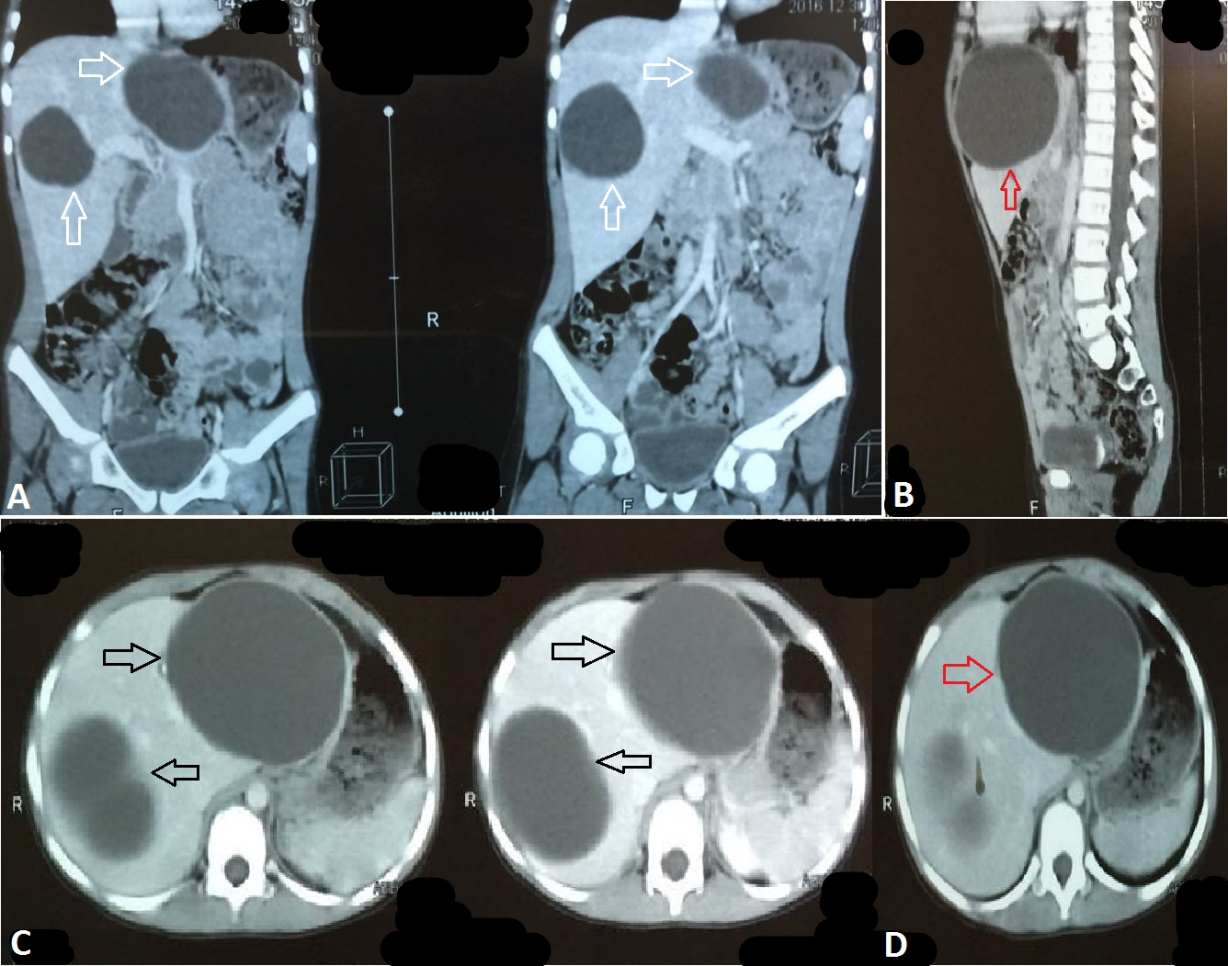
Computed Tomography Scan Findings Computed tomography (CT) scan showing two enlarged cysts in frontal view (white arrows in A). Massive cyst (red arrows in B and D). Two enlarged cysts in cross sectional view (black arrows in C).
